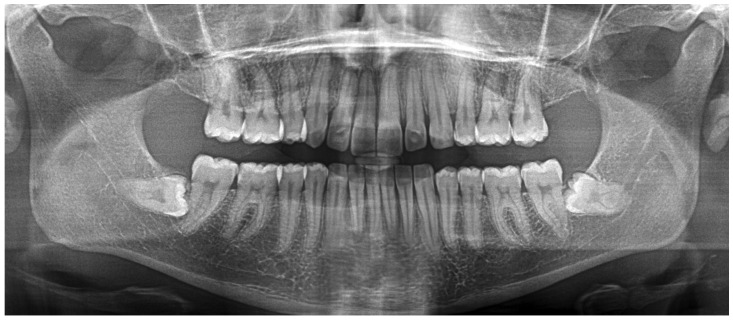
Final panoramic radiograph (prior to restorative procedures).
